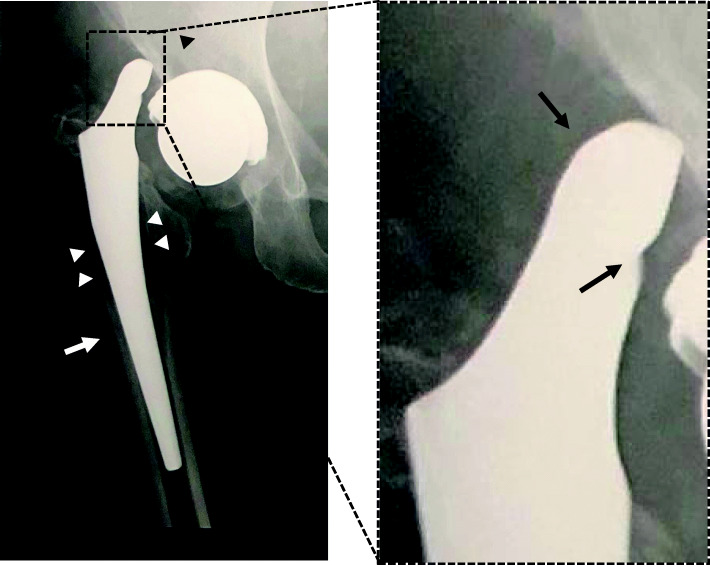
Radiography obtained 7.5 years after total hip arthroplasty (THA). There is dissociation of the femoral head from the head-stem trunnion (right panel, box) after THA (right panel, black arrows), radiolucency (left panel: black arrowhead, and white arrowheads), and cortical hypertrophy (white arrow)
